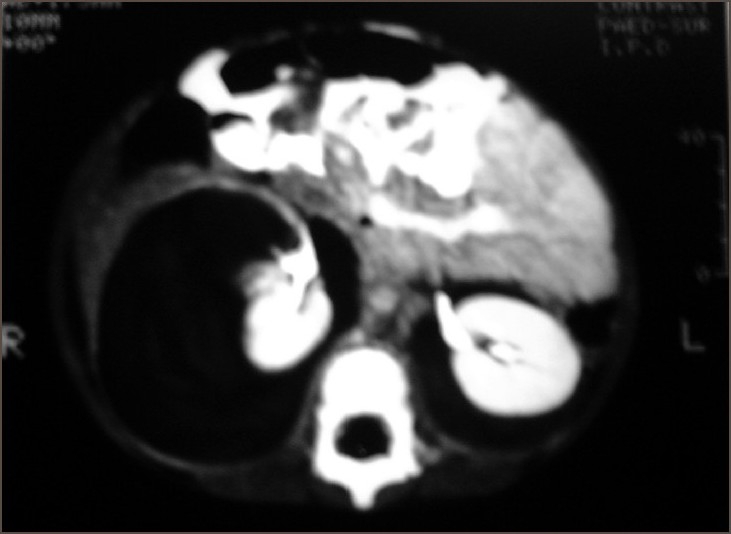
CT scan showing right renal hematoma
